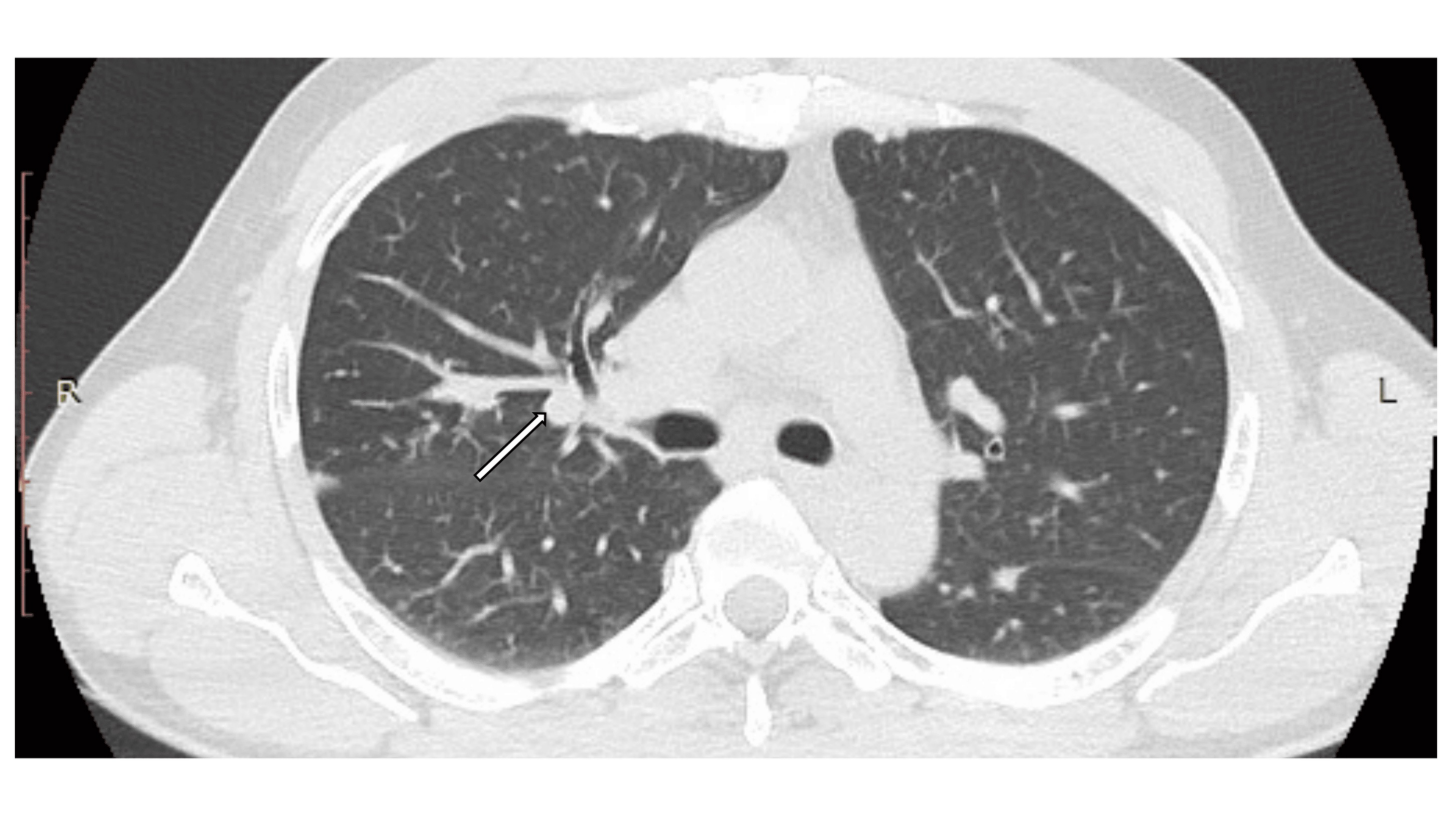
High-resolution CT of the chest. Centrilobular nodules in perilymphatic and subpleural distribution in both lungs with paratracheal lymphadenopathy, suggestive of grade 3 pulmonary sarcoidosis (arrow).
